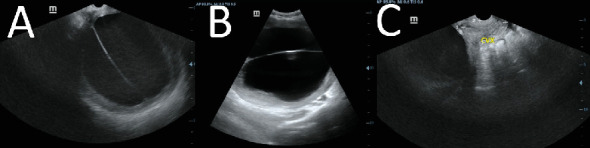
Initial ultrasound examination of the pelvis on the sagittal (A) and axial (B) images showed a large, well-defined cystic mass lesion in the pelvis with internal septations. The uterus and adnexa are not well visualized on scanning with only the laterally displaced cervix was visualized (C).
